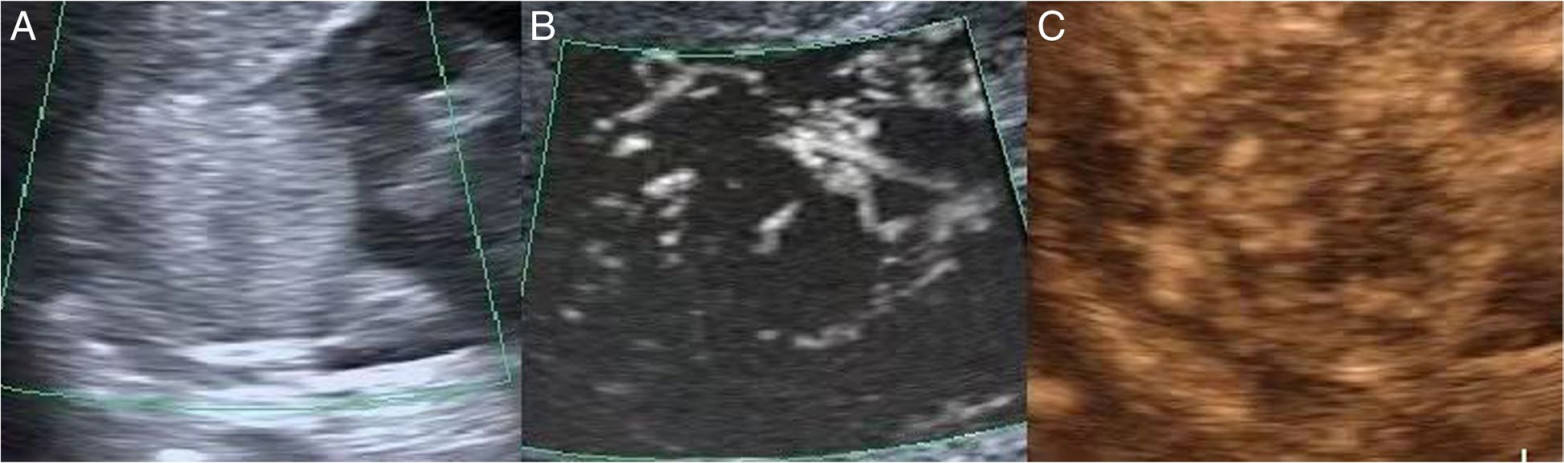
A solid tumor of the right kidney A ultrasound imaging, hyperechoic mass with clear boundary, regular shape, and inhomogeneous internal echo B SMI show a small blood vessels can be seen in the mass, the blood vessel architecture for type II C MFI showed peripheral and internal contorts the blood vessels, blood vessel vascular architecture for type III, pathological results of hamartoma
